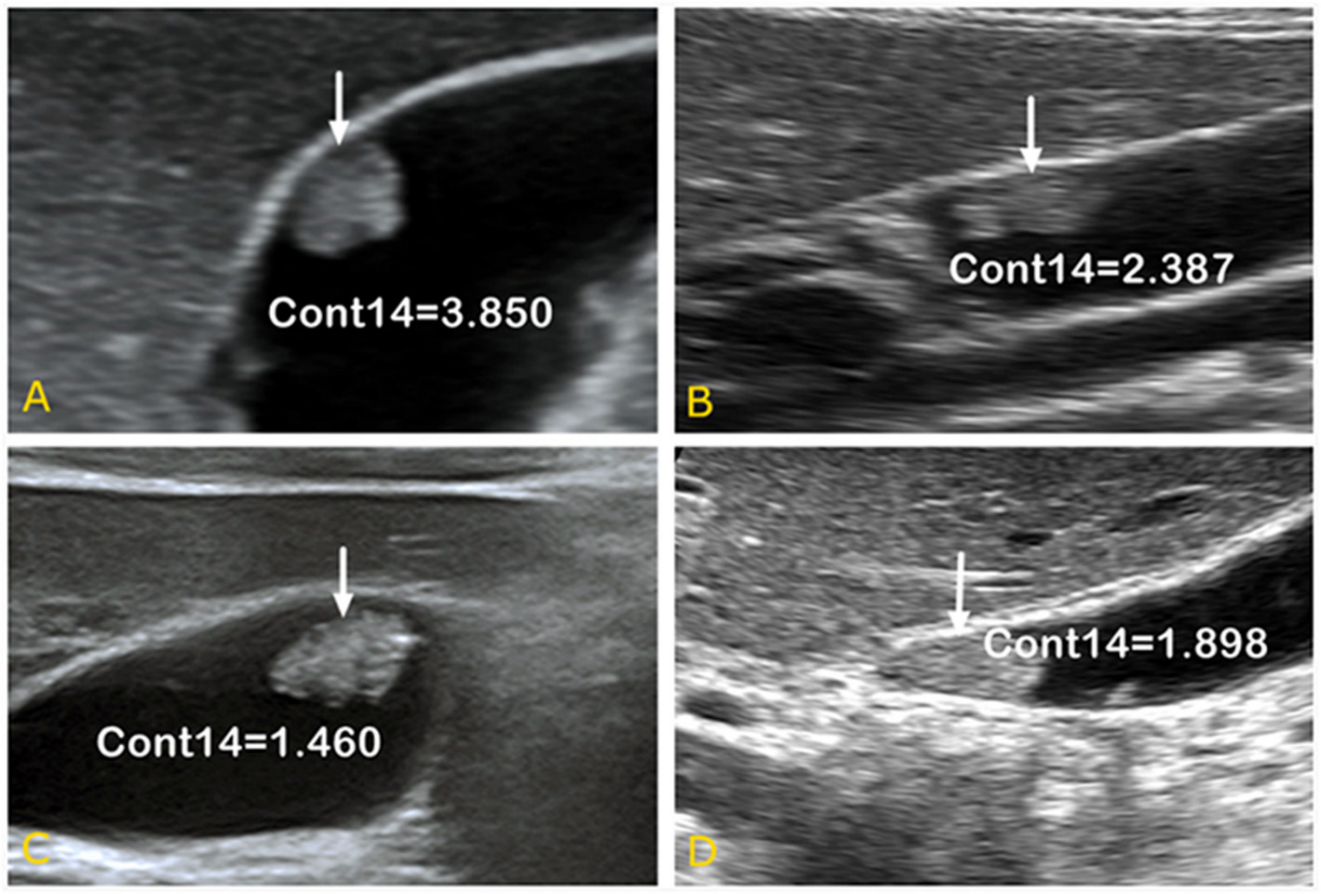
Ultrasound images of gallbladder cholesterol polyps (A,B) and gallbladder tubular adenomas (C,D). When combined with spatial parameter analysis, the Cont14 values were significantly higher (3.850 and 2.387) than those of the gallbladder adenomas (1.460 and 1.898), indicating the pixel distribution of the cholesterol polyp lesions was more uneven than that of the gallbladder tubular adenomas. Cont, contrast.
